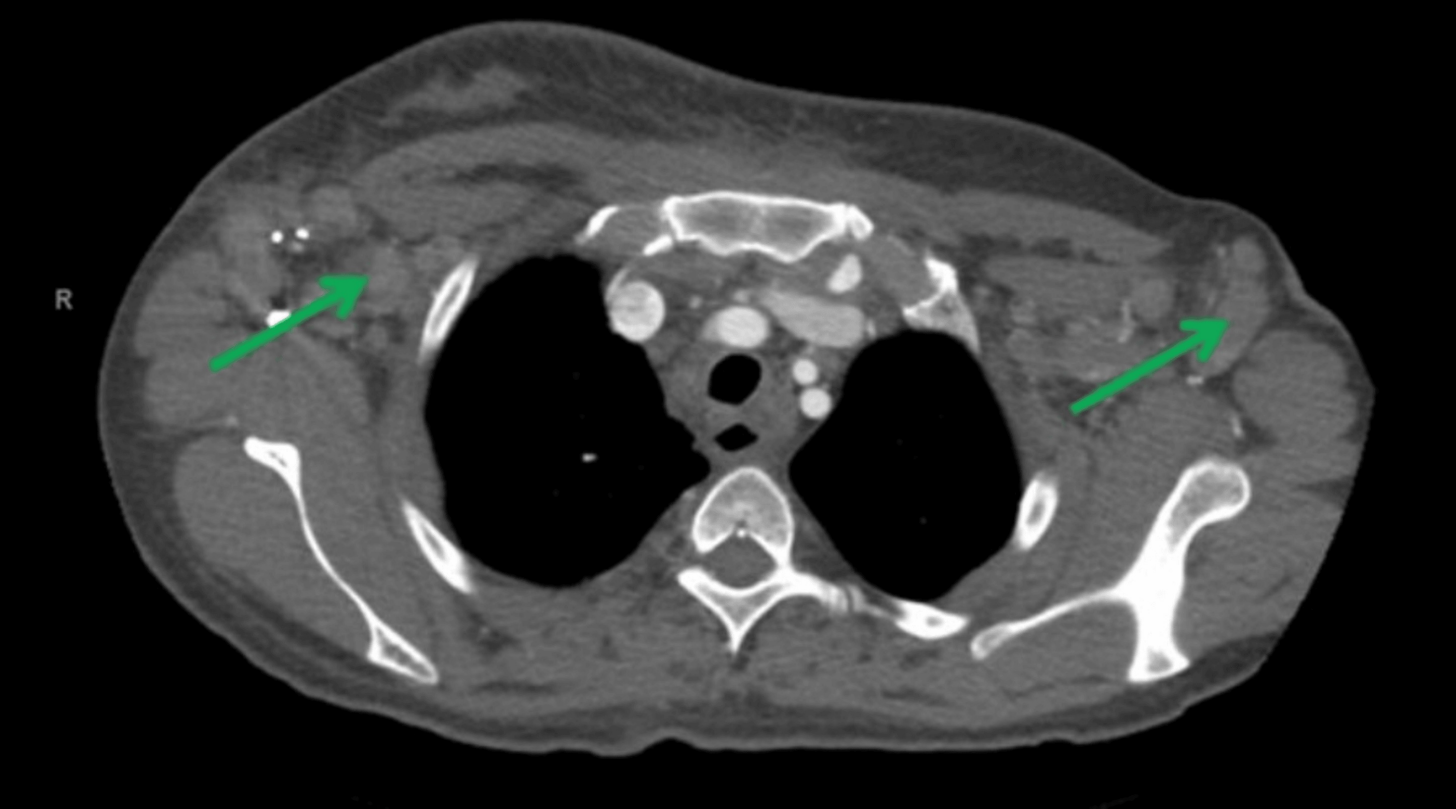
CT chest with contrast demonstrating axillary adenopathy (green arrows).
